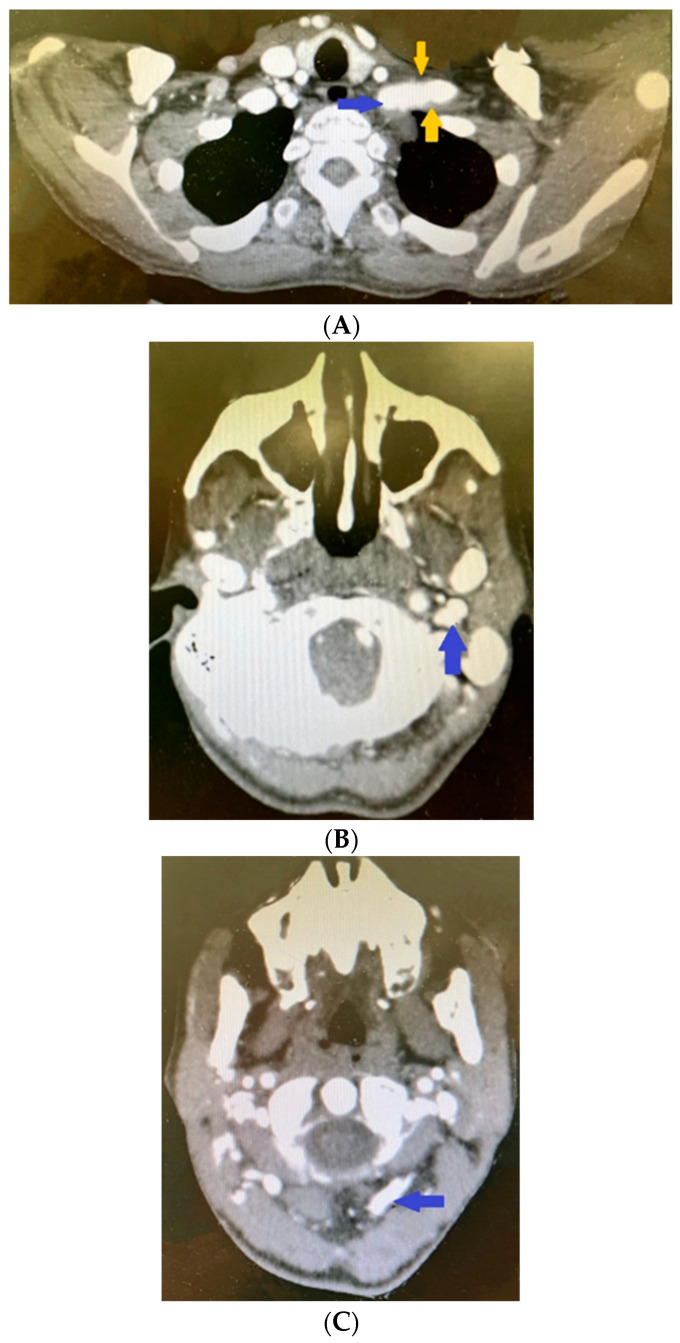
Contrast-enhanced computed tomography revealing (A) dilated left subclavian artery (blue arrow) and parietal thrombosis in the left subclavian artery (yellow arrows). Contrast-enhanced computed tomography revealing (B) aneurismal dilatation of left internal carotid artery. Contrast-enhanced computed tomography revealing (C) fusiform dilatation of left vertebral artery.
